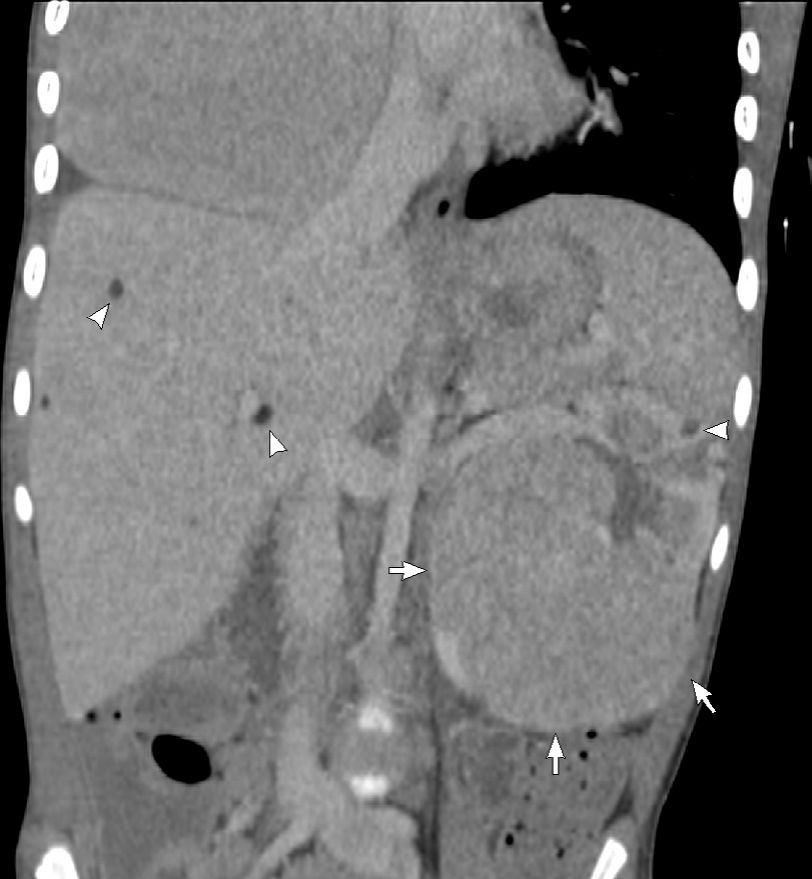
CECT of the abdomen in coronal reformat showing the exophytic left renal mass (arrows) causing mild mass effect upon left renal vein and partially visualized anterior mediastinal mass. Note the attenuation similarity of both masses. There are multiple small left renal AMLs and hepatic lipomas/AMLs(arrowheads). AML, angiomyolipoma; CECT, contrast-enhanced CT.
